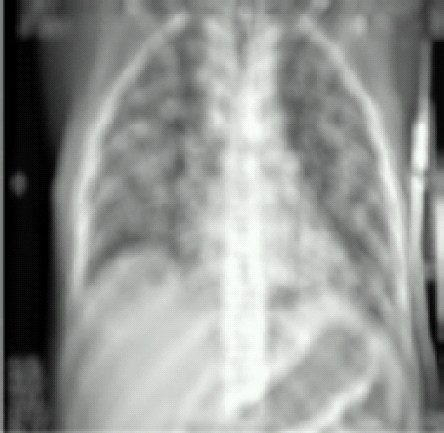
CT picture showing bilateral lung infiltrates
